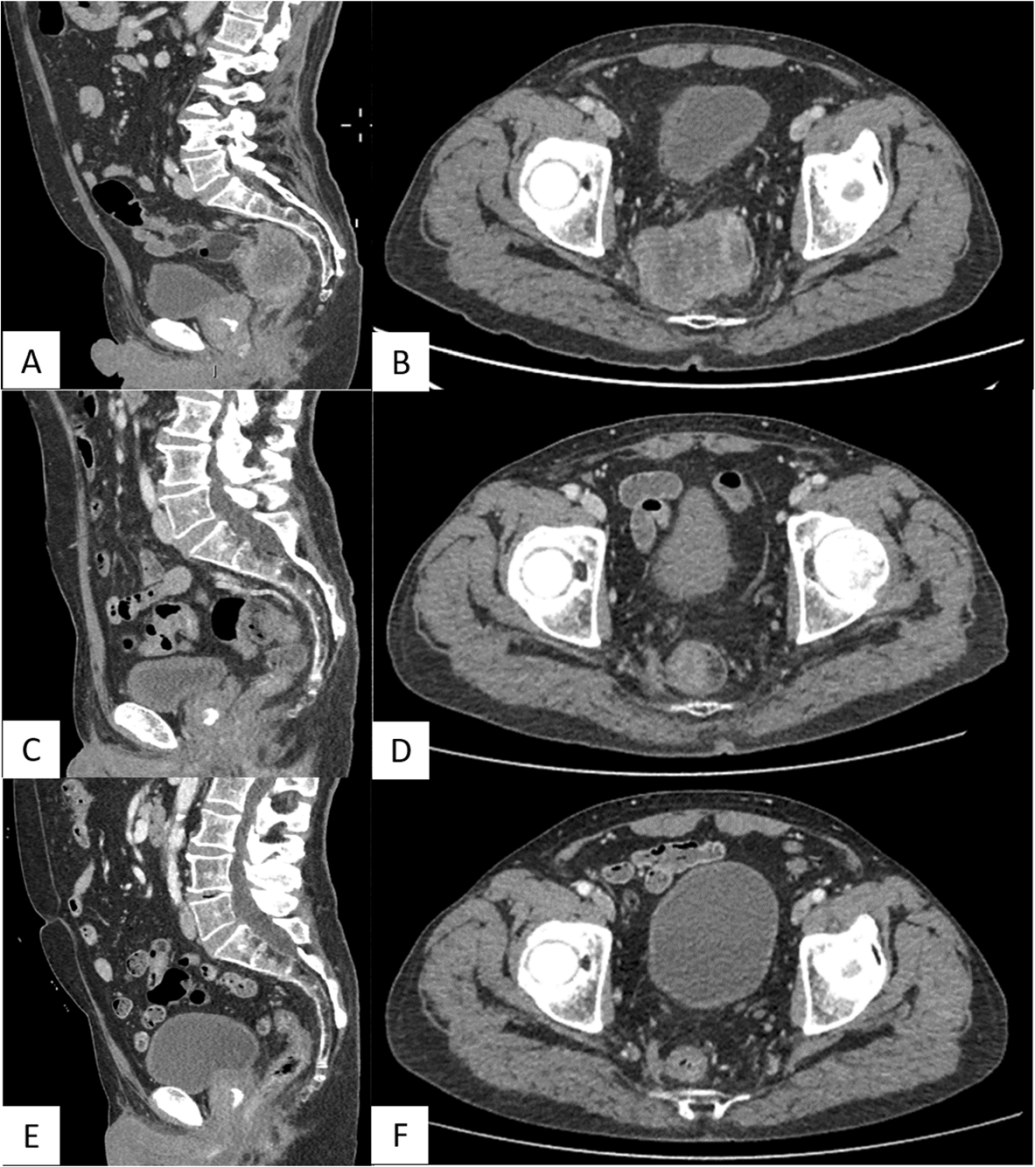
a, b CT scan showing a mass, centrally colliquated, originating from the right lateral wall of the rectum, infiltrating the right mesorectal fascia, the anterior presacral fascia, the homolateral piriformis muscle, and the right lobe of the prostate gland. The mass caused significant reduction in lumen calibre (a, sagittal plain. b, axial plain). c, d Re-evaluation of disease after the first three cycles of treatment. CT scan showed a marked reduction of the rectal mass of about 70–80%, with reduction also of lymph nodes and the prostatic involvement (c, sagittal plain. d, axial plain). e, f: CT evaluation after other three cycles of the same medical treatment. It showed further reduction of the rectal mass of about 50%. Lymph nodes and prostatic involvement disappeared (e, sagittal plain. f, axial plain)
